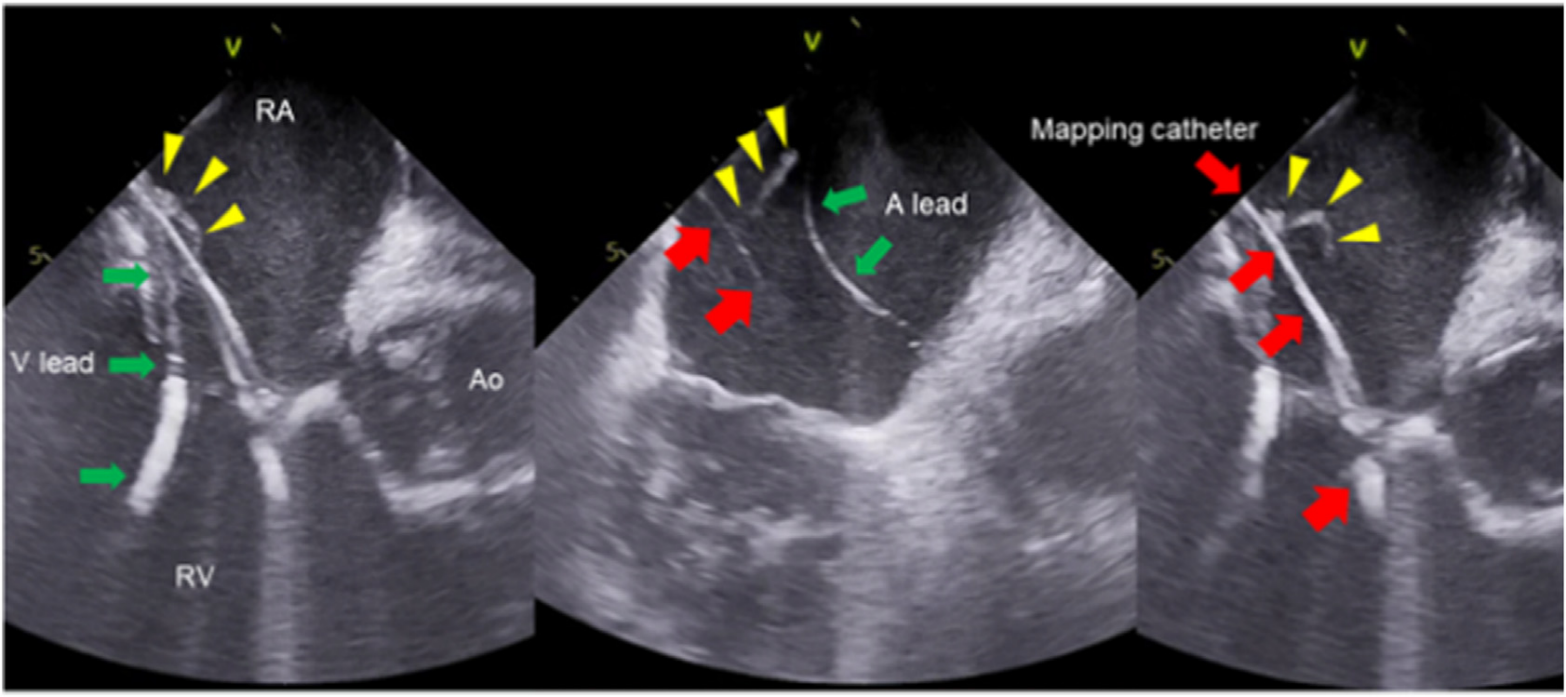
Consecutive images of the patient were recorded using intracardiac echocardiography. The yellow arrowheads indicate the thrombus. The red arrows indicate the mapping catheter. The green arrows indicate the ICD lead. A lead = atrial lead; Ao = aorta; RA = right atrium; RV = right ventricle; V lead = ventricular lead.
